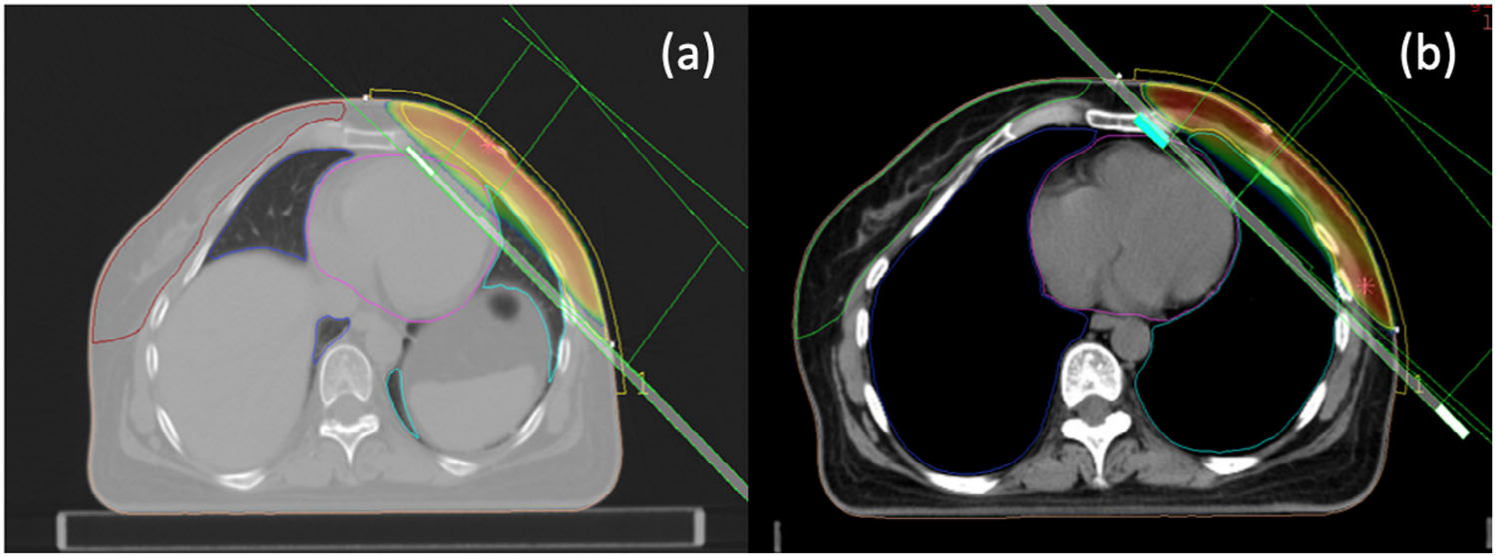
(a) Involvement of heart partial volume under radiation beam arrangement in free breathing CT (a): and vin DIBH CT.
